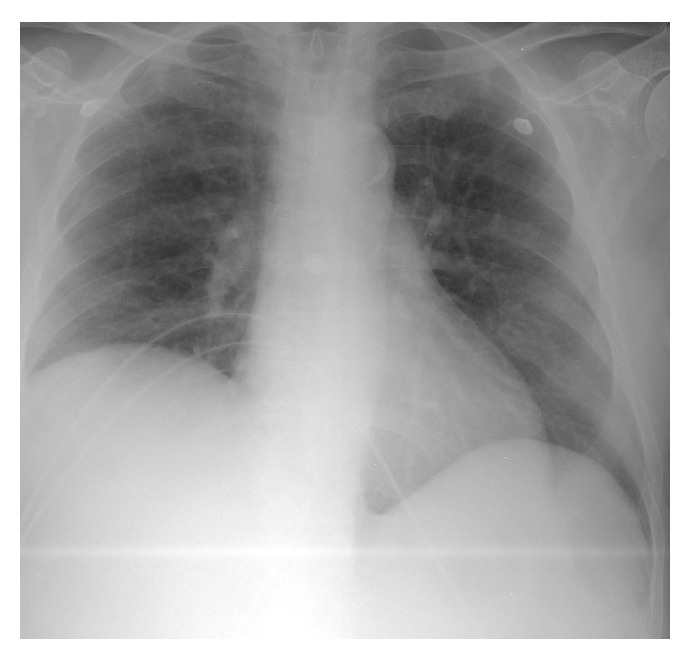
Portable chest X-ray. Showing elevation of right dome of the diaphragm. There is atherosclerotic calcification of aortic arch. The cardiac silhouette is within normal limits.
